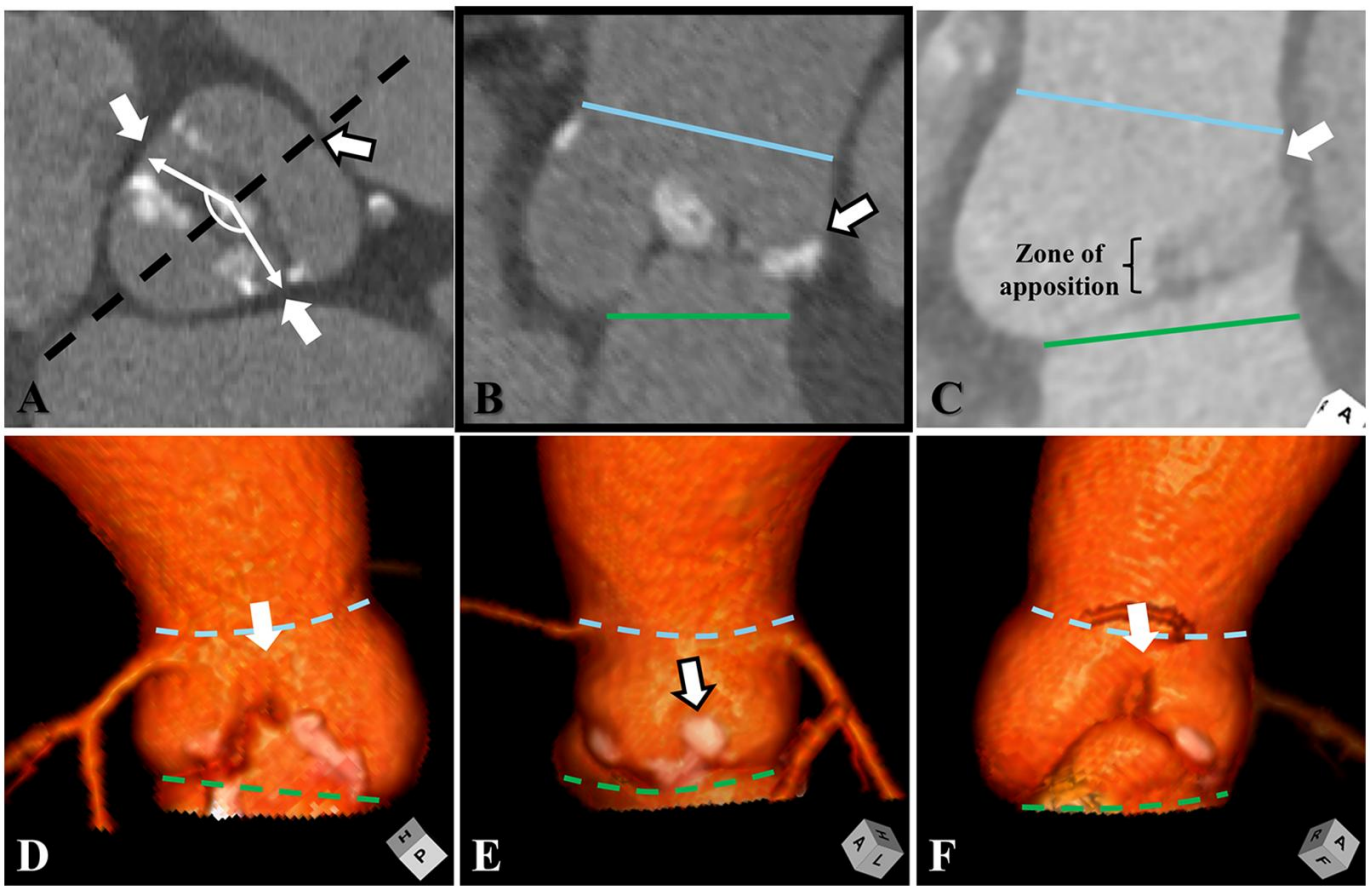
Cardiac computed tomographic assessment of the commissural angle and commissural heights are demonstrated. (A) Short axis of the aortic root is obtained to measure the commissural angle (angulated white double-headed arrow). In this functionally bileaflet aortic valve with fusion between the coronary leaflets it measures approximately 170 degrees. The black hashed line represents the center bisecting plane, which cuts from the interrogated commissure across to the midline of the opposite leaflet. (B) In the center bisecting plane, the height of the fused commissure (white arrow with black outline) above the plane of the virtual basal ring (green lines and hashed lines) will be short relative to the sinutubular junction (blue lines and hashed lines) and relative to interrogation of the normal commissures. (C) The long axis of the center bisecting plane is demonstrated in a normal trileaflet valve depicting a double shadow of the interrogated zone of apposition, leading up to the normal commissure (white arrow). (D–F) Three-dimensional reconstructions of the depicted bileaflet valve demonstrate three sinuses, with a shortened interleaflet triangle (white arrow with black outline) and commissural height of the fused coronary leaflets relative to the other two normal commissures (white arrow).
